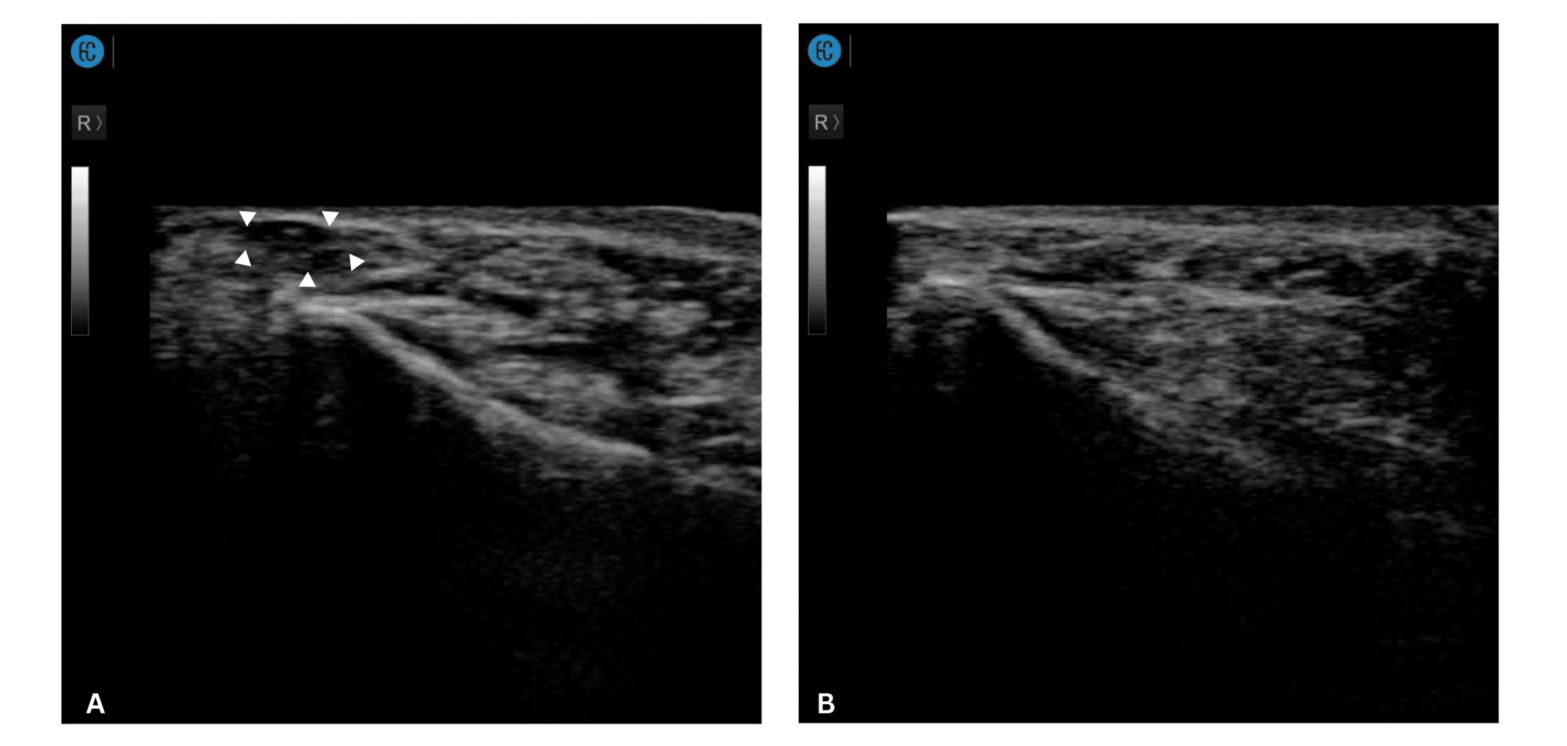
Ultrasonographic appearance of the tear trough filler nodule before and after treatment (Case 2) (A) Ultrasound image of the right infraorbital region in the oblique plane demonstrating a well-defined hypoechoic lesion (indicated by arrows) within the superficial subcutaneous layer, consistent with aggregated filler material. (B) Follow-up ultrasound image of the right infraorbital region obtained one week post-treatment, showing complete resolution of the previously observed hypoechoic lesion.
